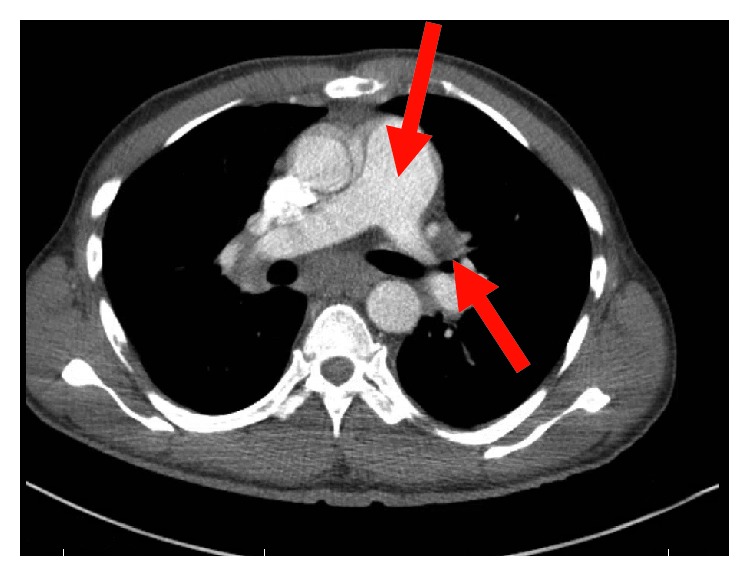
CT chest with contrast showing enlarged pulmonary artery, mediastinal, and hilar lymphadenopathy.
